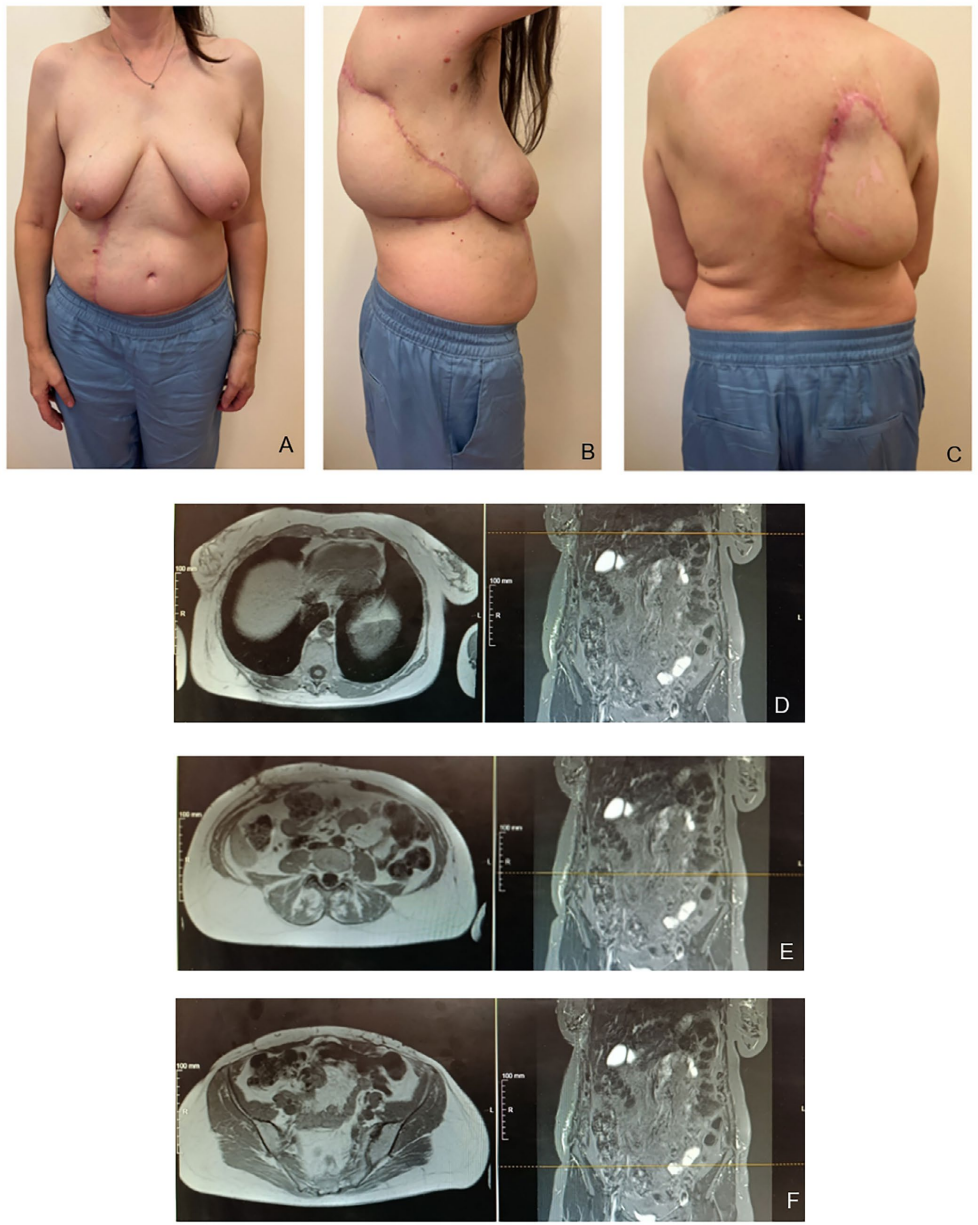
Postoperation pictures at 6 months, respectively, anterior (A), lateral (B), and posterior (C), and iodine contrast‐MRI multiple imaging of trunk (D), abdomen (E), and pelvis (F).
